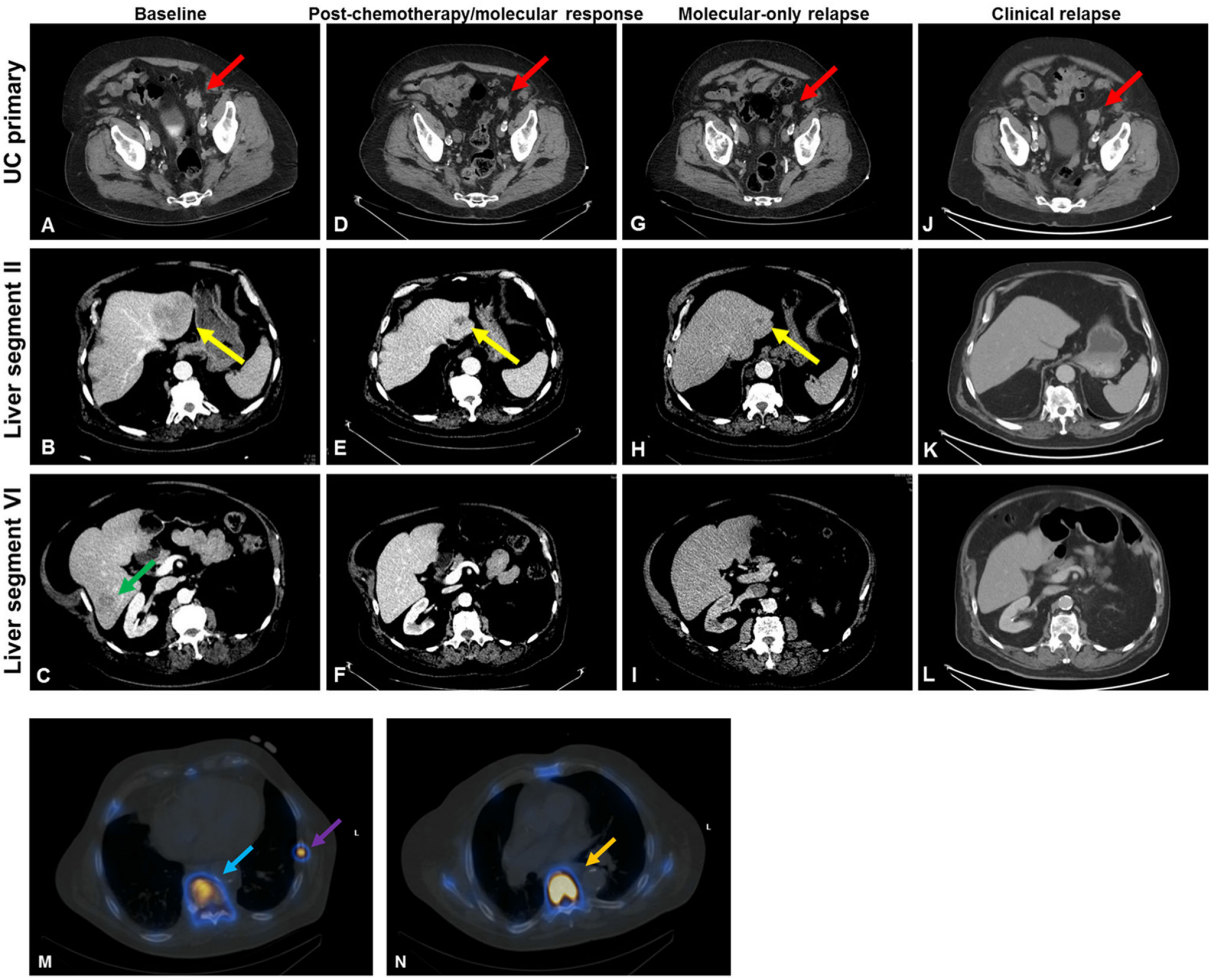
Radiographic response of mUC to treatment in our patient. A–C Pre-treatment CT demonstrated UC primary mass measuring 2.9 × 2.7 cm ((A) red arrow), liver segment II metastasis measuring 7.3 × 6.4 cm ((B) yellow arrow), and liver segment VI metastasis measuring 2.3 × 2.4 cm ((C) green arrow). At this timepoint, our post-hoc ctDNA analysis detected TP53 G245D at 42.9% VAF, BRCA1 p.N1521fs at 47.3% VAF, and 429 FGFR3ex18-TACC3ex12 fusion reads (normalized to a reference of 6089 total FGFR3ex18 reads). D–F CT scan after 3 cycles of cisplatin/gemcitabine chemotherapy showed minimal response in the UC primary mass measuring 2.3 × 2.1 cm ((A) red arrow); partial response seen in liver segment II metastasis now measuring 1.8 × 2.1 cm ((E) yellow arrow) and resolution of liver segment VI metastasis (F). At this timepoint, ctDNA abnormalities were no longer detectable by sequencing vendor’s clinical reports, although on post-hoc analysis certain abnormalities were still detectable at low VAF as detailed in Fig. 1B and Supplemental Table 2. G–I CT scan at time of molecular relapse (by sequencing vendor’s clinical reports) shows stability of UC primary measuring 2.1 × 2.1 cm ((G), red arrow), and ongoing radiographic response in liver segment II metastasis measuring 1.3 × 1.6 cm ((H) yellow arrow) and continued resolution of liver segment VI metastasis (I). At this timepoint, our post-hoc ctDNA analysis detected TP53 G245D at 0.26% VAF, BRCA1 p.N1521fs at 0.49% VAF, and 2 FGFR3ex18-TACC3ex12 fusion reads (normalized to a reference of 4513 total FGFR3ex18 reads). J–L CT scan at time of frank clinical relapse with progression of the UC primary mass, now measuring 2.5 × 2.4 cm ((J) red arrow). Liver segment II and VI metastases (K, L) are not seen. However, multifocal bone progression is better appreciated on bone scintigraphy (M, N). At this timepoint, our post-hoc ctDNA analysis detected TP53 G245D at 49.1% VAF, BRCA1 p.N1521fs at 54.7% VAF, and 1238 FGFR3ex18-TACC3ex12 fusion reads (normalized to a reference of 8890 total FGFR3ex18 reads). M, N Bone scintigraphy at time of clinical progression. M. Bone scintigraphy demonstrating a new T9 sclerotic lesion (blue arrow) demonstrating Tc-99m MDP uptake consistent with a bony metastasis. Biopsy subsequently demonstrated metastatic UC (Fig. 3). Also shown is an additional area of Tc-99m MDP uptake at the left ninth rib (purple arrow), also consistent with bony metastasis. N Additional area of Tc-99m MDP uptake at T7 (orange arrow), consistent with bony metastasis. In addition to T7, T9, and left ninth rib lesions shown above, bone scan also demonstrated abnormal radiotracer uptake suspicious for metastatic disease at T3, T8, T10, right orbit, sternum, right second rib, and proximal femurs (not shown).
